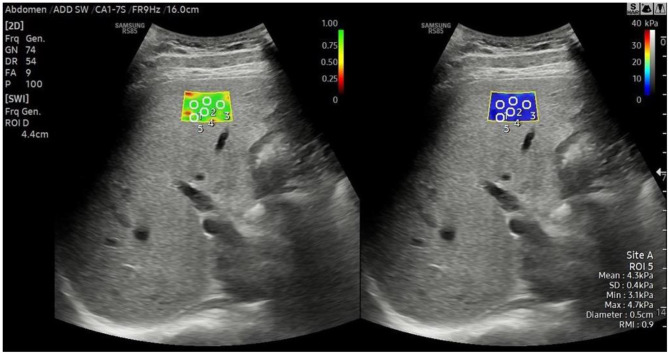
Example of a 15 year-old female underwent 2D shear wave ultrasound of the liver (Samsung prestige RS85 - Samsung Medison, Seoul, South Korea) with multiple measurements expressed as kPa
